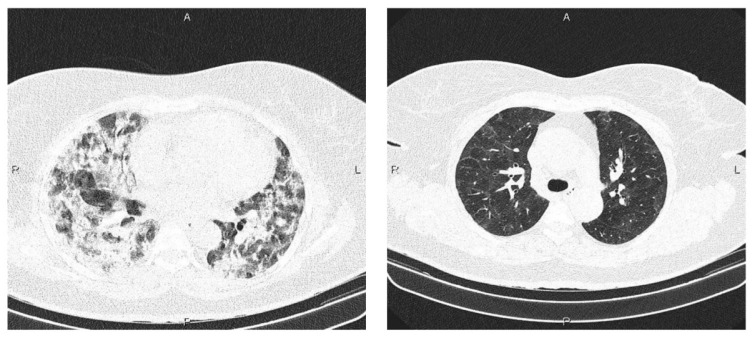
CT scan image data: Example image of COVID-19 positive CT scan (left) and non-COVID-19 CT scan (right) (courtesy: radiopaedia.org, rID: 85896).
